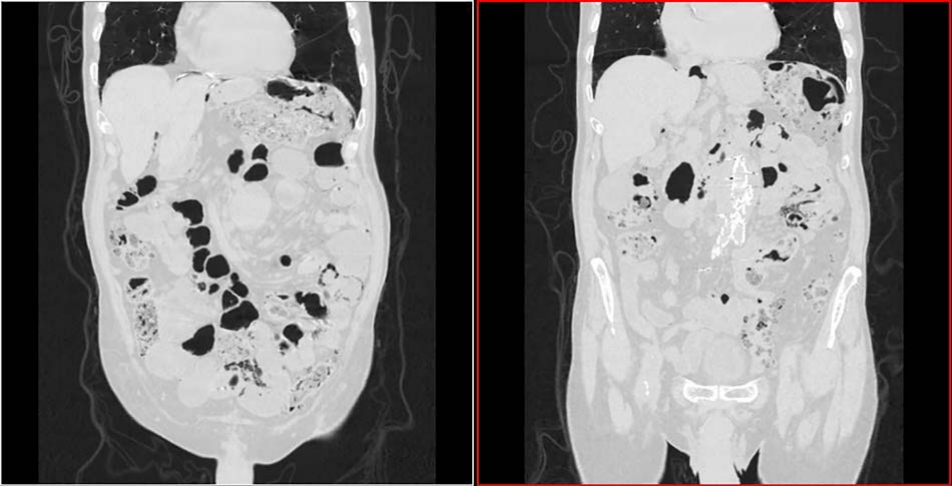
Transversal CT image in lung window showing pneumoperitoneum and pneumatosis intestinalis.
